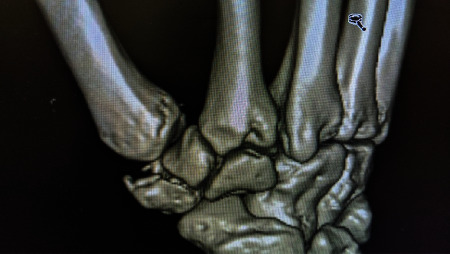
CT with 3D reconstruction detailing trapezium fracture.
